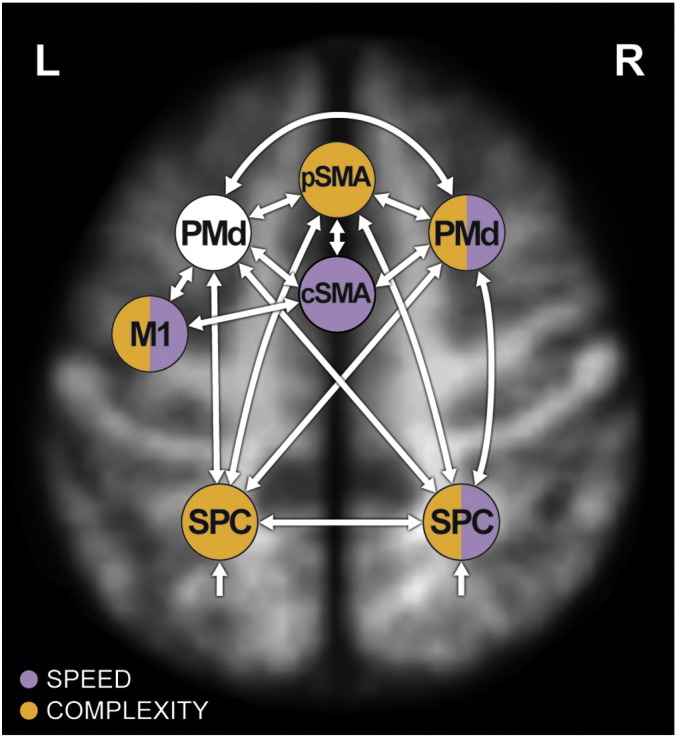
Dynamic Causal Model specified in each participant. White arrows represent condition-independent connections between regions of interest. Modulations of these connections by experimental factors, i.e. condition-dependent modulations, are colour-coded (purple for speed, orange for complexity modulation). The colour of the region represents the modulation by the respective experimental factor(s). All afferent connections from other regions towards the respective region are modulated. All specified model parameters can be reviewed in Supplement 3. For reasons of figure legibility, anatomical location of regions is approximated. The model is superimposed on a mean T1-weighted structural image of all participants, normalised to MNI space.
